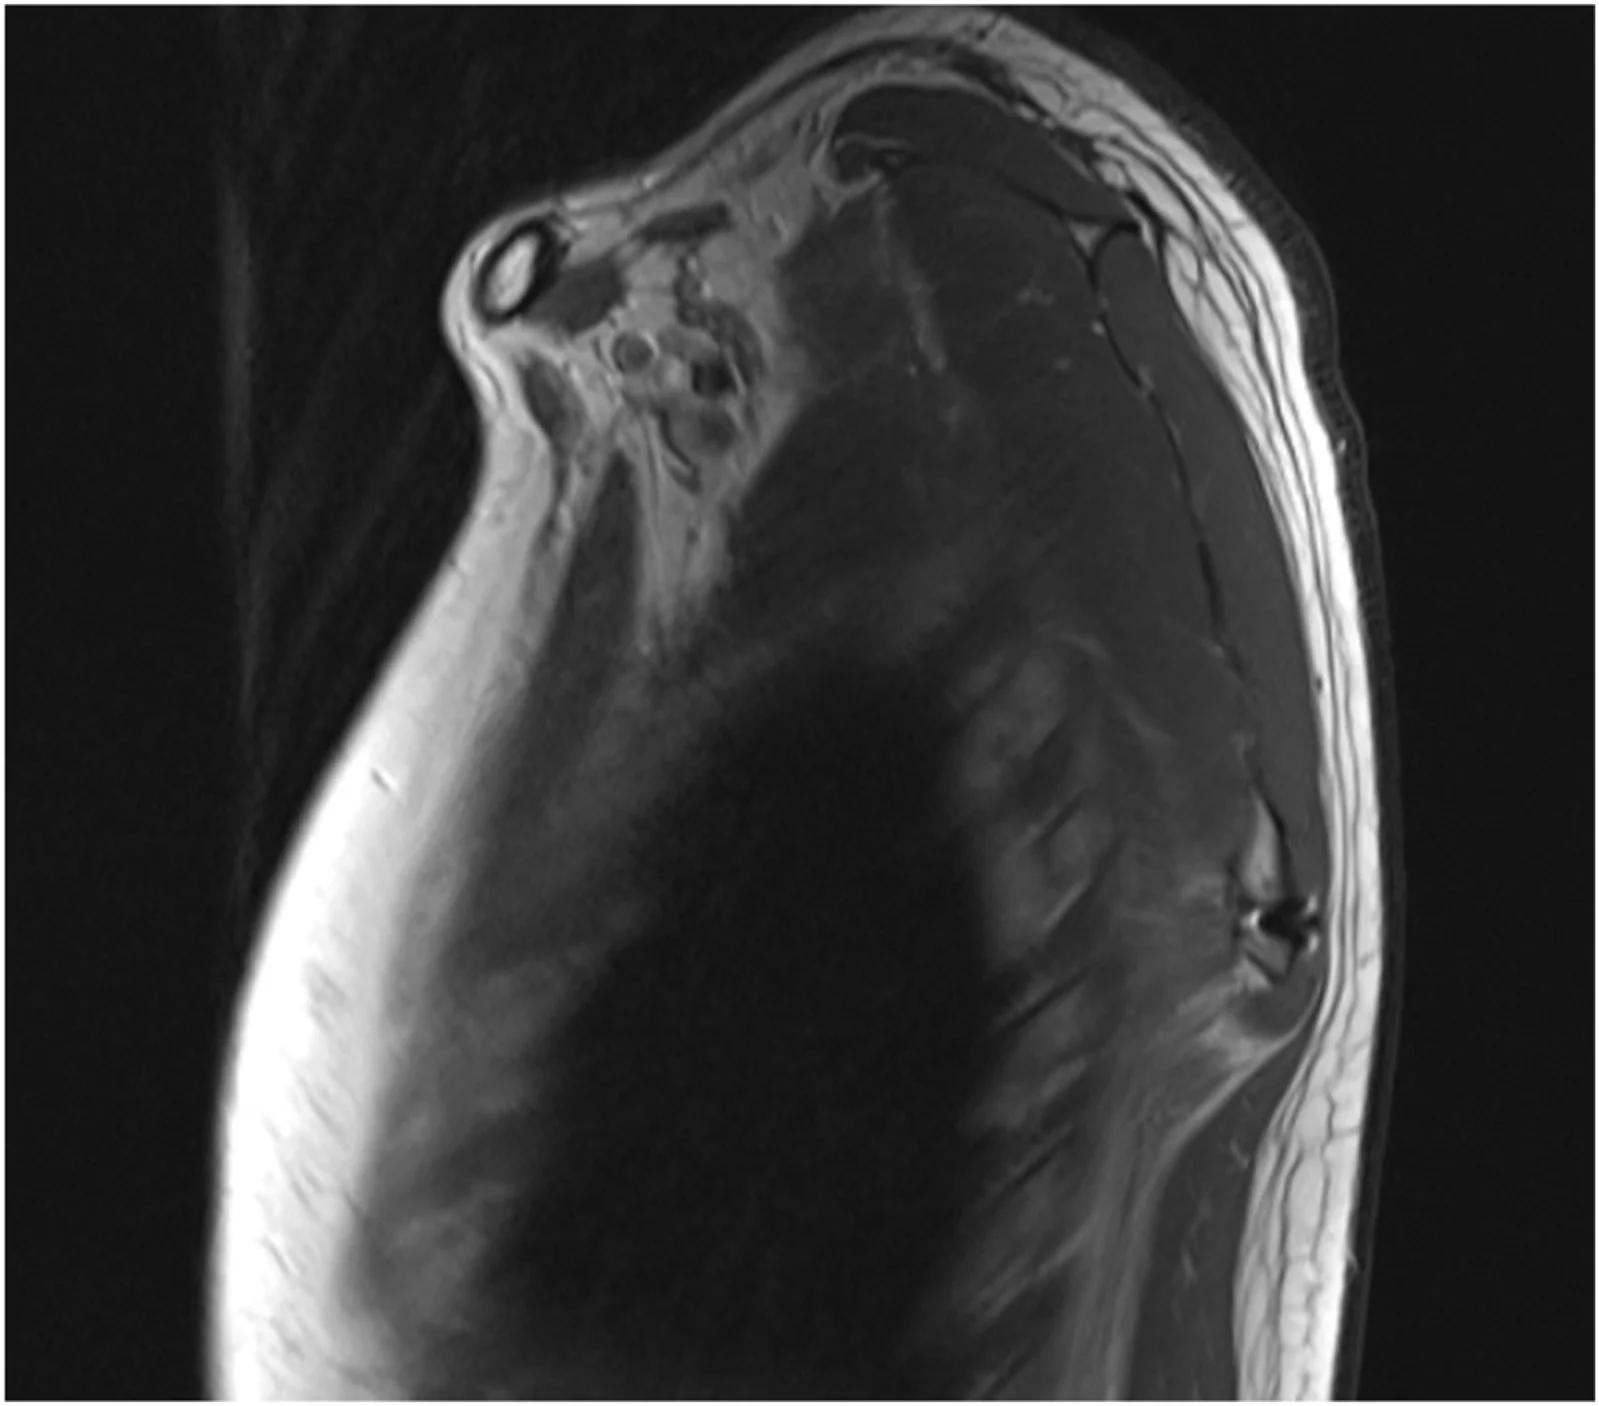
Sagittal T1-weighted magnetic resonance image of the thorax demonstrating the cortical button positioned at the inferior angle of the scapula following suspensory fixation.
